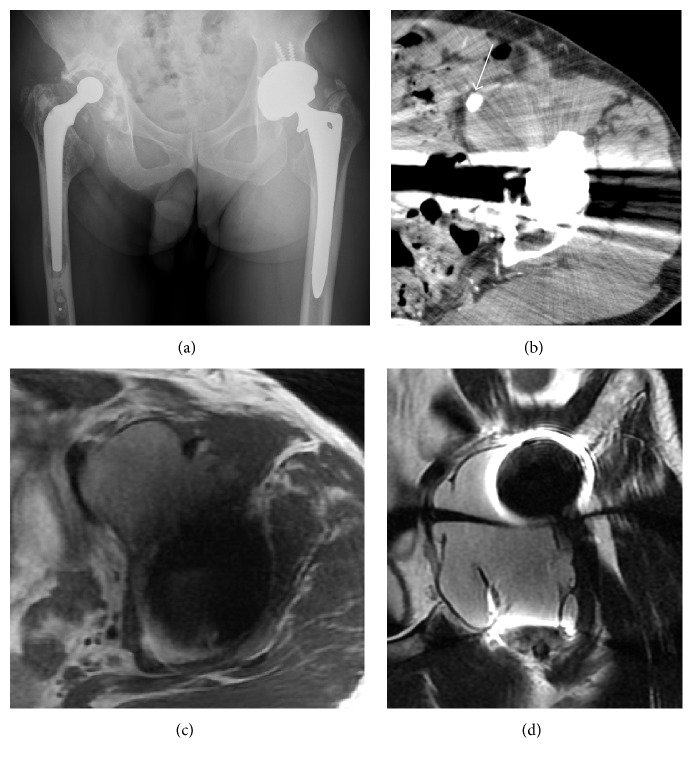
Plain radiographic, contrast-enhanced computed tomographic, and magnetic resonance images of the hip. Anteroposterior view on plain radiography shows no abnormal findings around the left hip (a). Axial view on contrast-enhanced computed tomography reveals a cystic lesion at the anterior aspect of the left hip compressing the left femoral vessels (white arrow, (b)). Magnetic resonance imaging reveals a cystic lesion extending from the anterior aspect of the left hip to the intrapelvic region. The mass has iso-high intensity on a T1-weighted axial image (c) and high intensity on a T2-weighted coronal image (d).
